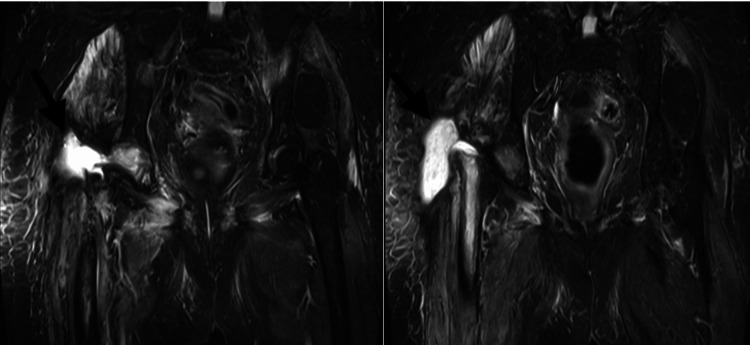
MRI showing fluid retention MRI: magnetic resonance imaging
